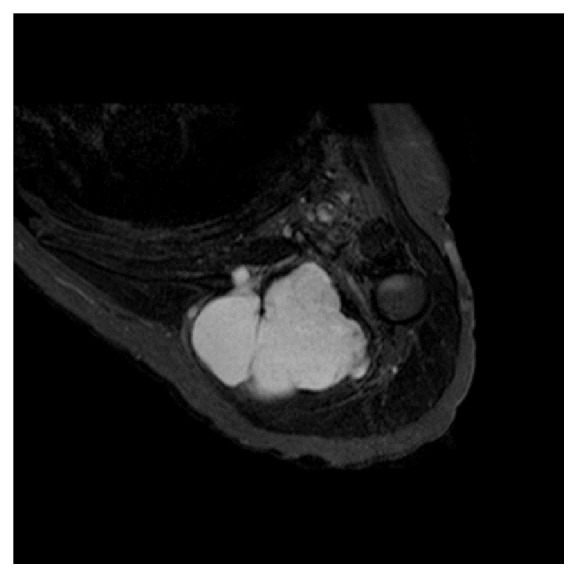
STIR and T2-weighted transverse MRI. Observe the huge cystic-like hyperintense mass growing inside the deltoid muscle and invading the scapula. The mass contains some septations but there is no apparent solid component.
